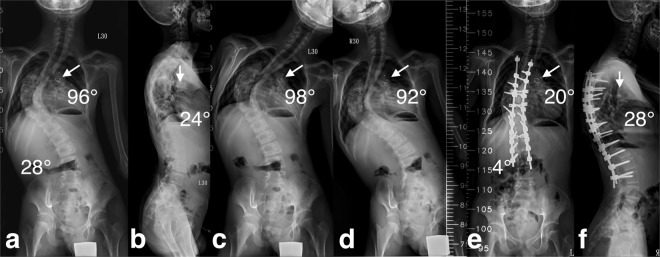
A 16-year-old boy had underlying diseases of TOF and severe AIS. The preoperative radiographs showed severe scoliosis with limited flexibility, and postoperative radiographs showed significant improvements in the deformities. The pulmonary stent and Cobb angles of each curve were designated. (a) Preoperative AP radiograph; (b) Preoperative lateral radiograph; (c,d) Preoperative left and right bending films; (e) AP radiograph at latest follow-up; (f) Lateral radiograph at latest follow-up.
